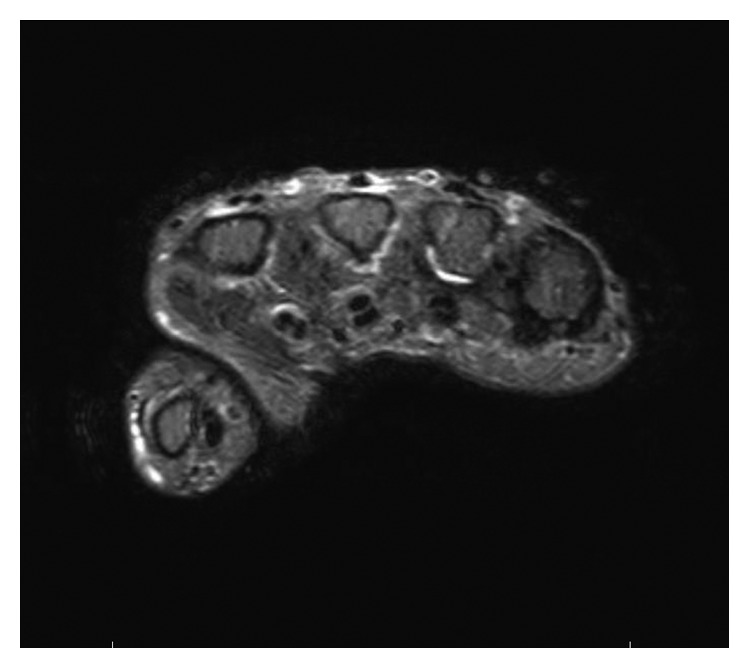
MRI of the right hand without contrast. Short T inversion recovery (STIR) showing tenosynovitis of the flexor tendons of the second and third digits and synovitis of the second, third, and fourth MCP joints.
